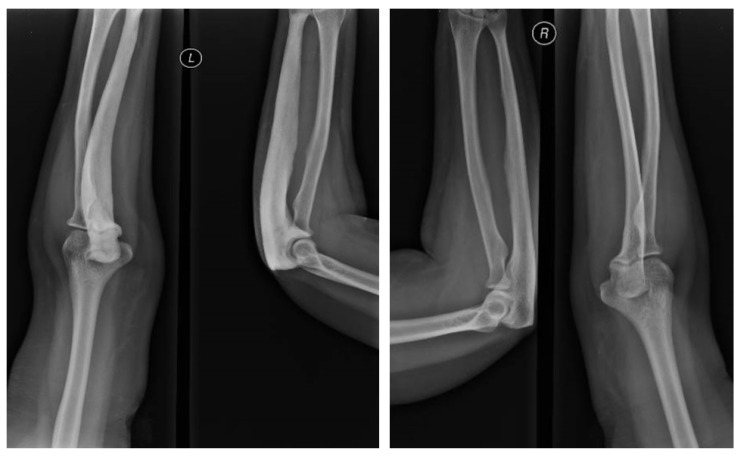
X-ray examination: Enlargement of the proximal left ulna and cortical thickening, suggestive of PBD (X-ray of the left (L) and right (R) forearm).
